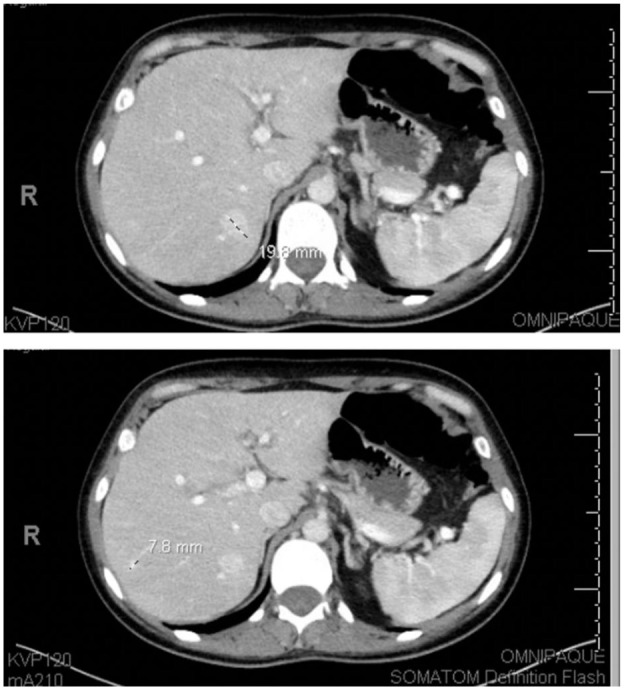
Initial presentation workup: CT abdomen pelvis showing two 19.8 and 7.8 mm right hepatic lobe enhancing lesions. Lesions were thought to represent a benign process, including hemangioma adenoma/FNH.
